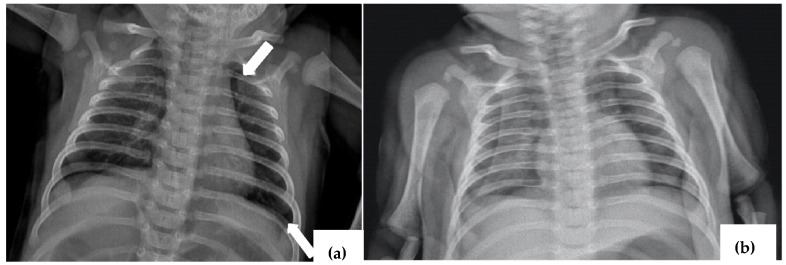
Thoracic X-ray (anterior–posterior) showing with arrows (a) small left pneumothorax; (b) lung re-expansion.
